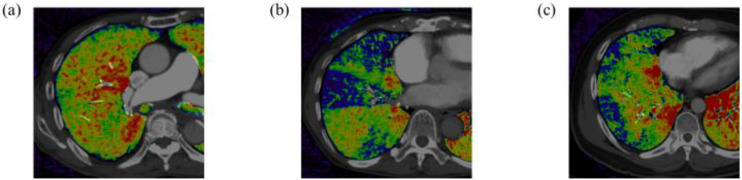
Horizontal section of DE-CT image in a patient with normal subpleural perfusion (a), wedge-shaped segmental defect (b), and poor subpleural perfusion (c). Black-blue color-coded image represents poor lung perfusion, and red-yellow color-coded image represents well lung perfusion.
